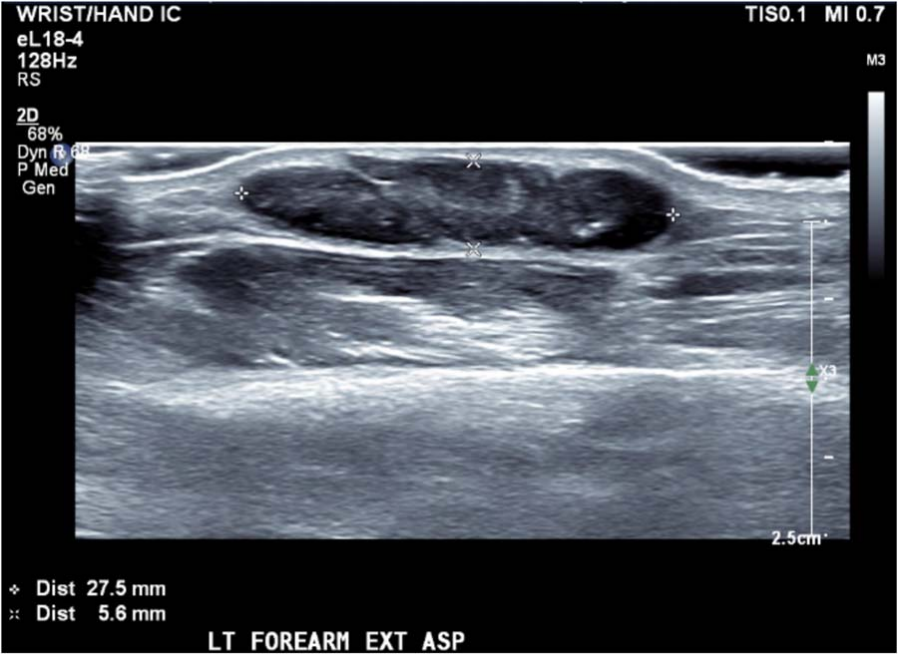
Ultrasound of left forearm with region of interest.
